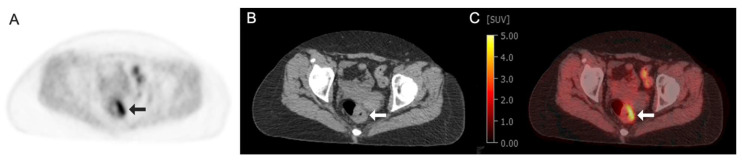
Images obtained from a 68-year-old man with rectal cancer demonstrating focal circumferential radiotracer uptake in left rectal wall thickening (arrows) at the site of primary tumor: (A) Fluorine 18 (^18^F)-labeled Fluoromisonidazole (FMISO) axial PET image; (B) axial CT image; (C) axial fused ^18^F-FMISO PET/CT image with an SUV max of 5.4.
